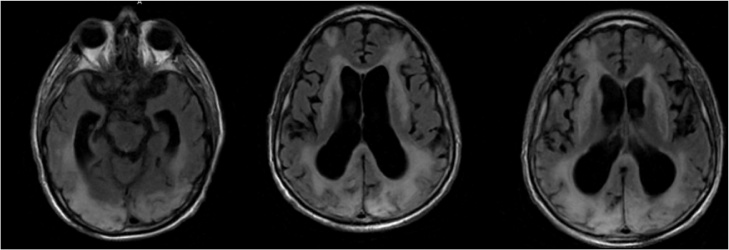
MRI showing extension stable white matter disease without evidence of acute infarct.
